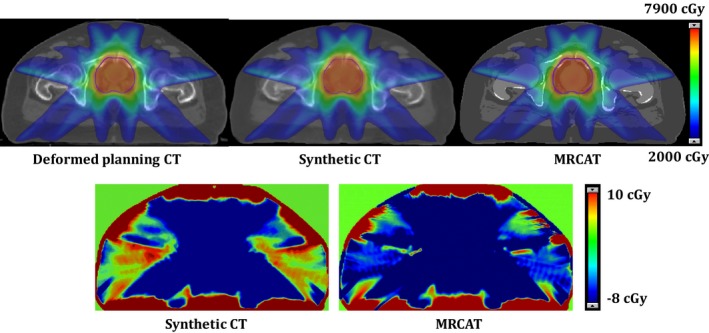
Example of dose distribution (Top row) for plans calculated using deformed planning CT, synthetic CT, and MRCAT CT along with dose difference maps between the deformed planning CT and the synthetic CT and MRCAT CT respectively.
